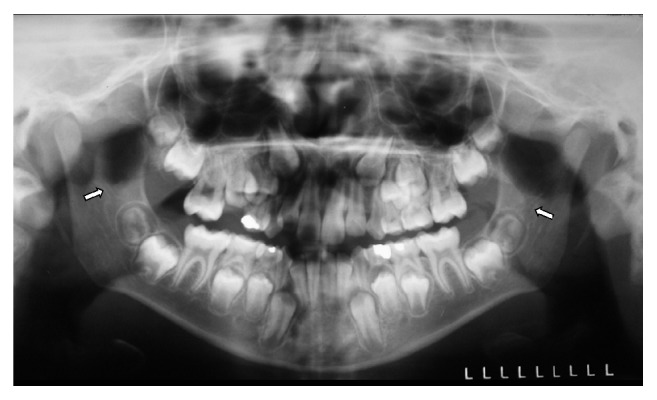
RMC (arrows) observed bilaterally located close to the mandibular third molar germs in a nine-year-old male patient in panoramic radiography.
